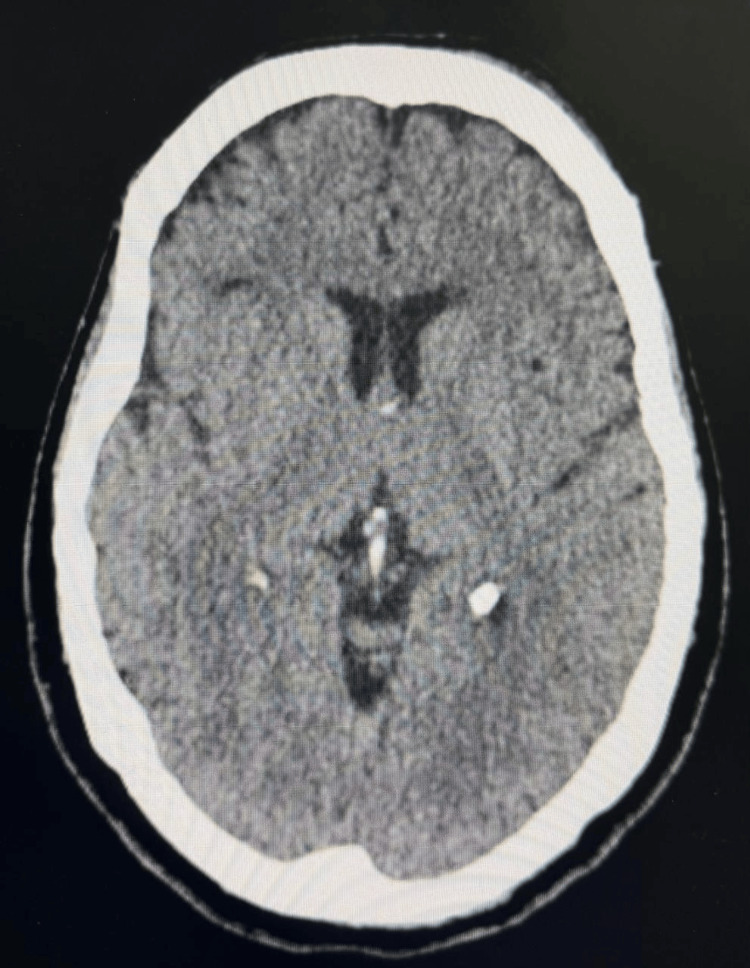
Non-contrast CT of the brain. Axial non-contrast CT scan shows preserved gray-white matter differentiation and symmetric ventricular configuration, with no signs of acute hemorrhage, mass effect, or ischemia. Despite significant neuropsychiatric manifestations, structural neuroimaging may remain unremarkable in early disease stages.
